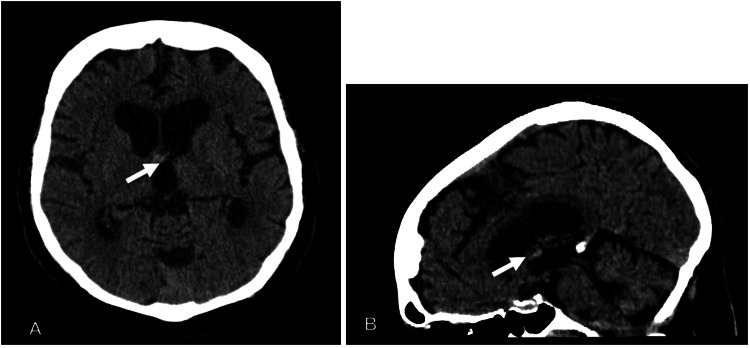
A hyperdense colloid cyst (white arrow) that was missed on the finalized interpretation report (A) CT head axial of study. (B) CT head sagittal of study.
